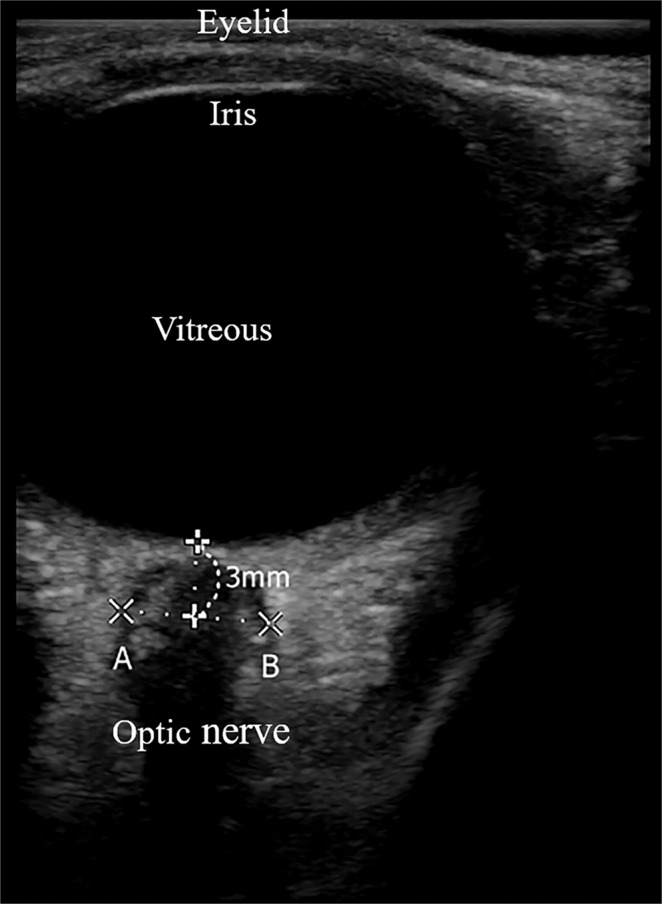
Measurement of optic nerve sheath diameter using ultrasonography. Axial plane images of the orbit were acquired through the optic nerve. Optic nerve sheath diameters were measured 3 mm posterior to the globe (A,B).
